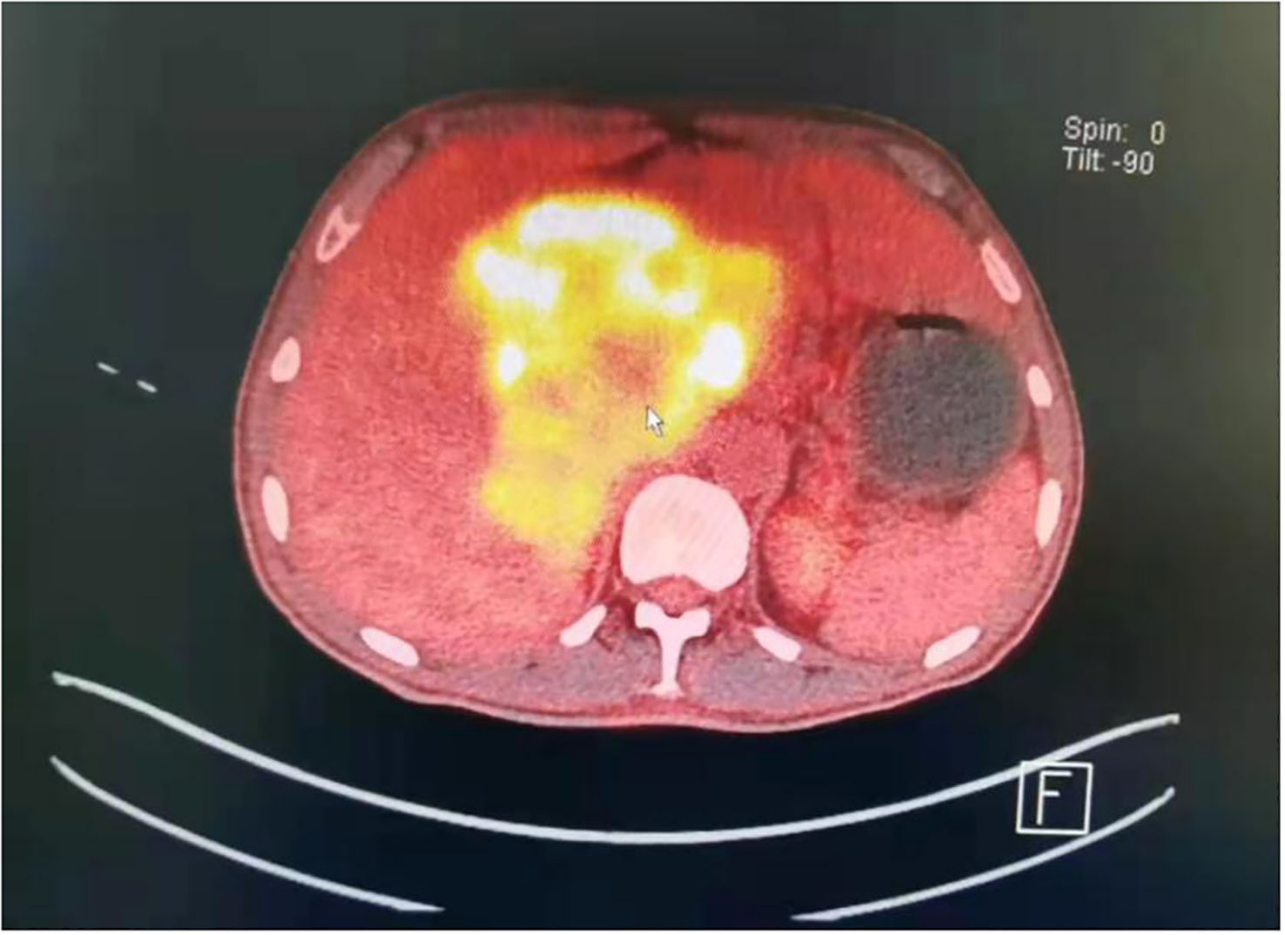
PET-CT, The paraganglioma was in the abdominal and retroperitoneal (liver and pancreatic) area. The interface size of the mass was approximately 148 mm * 100 mm, and the upper and lower diameter was approximately 155 mm.
